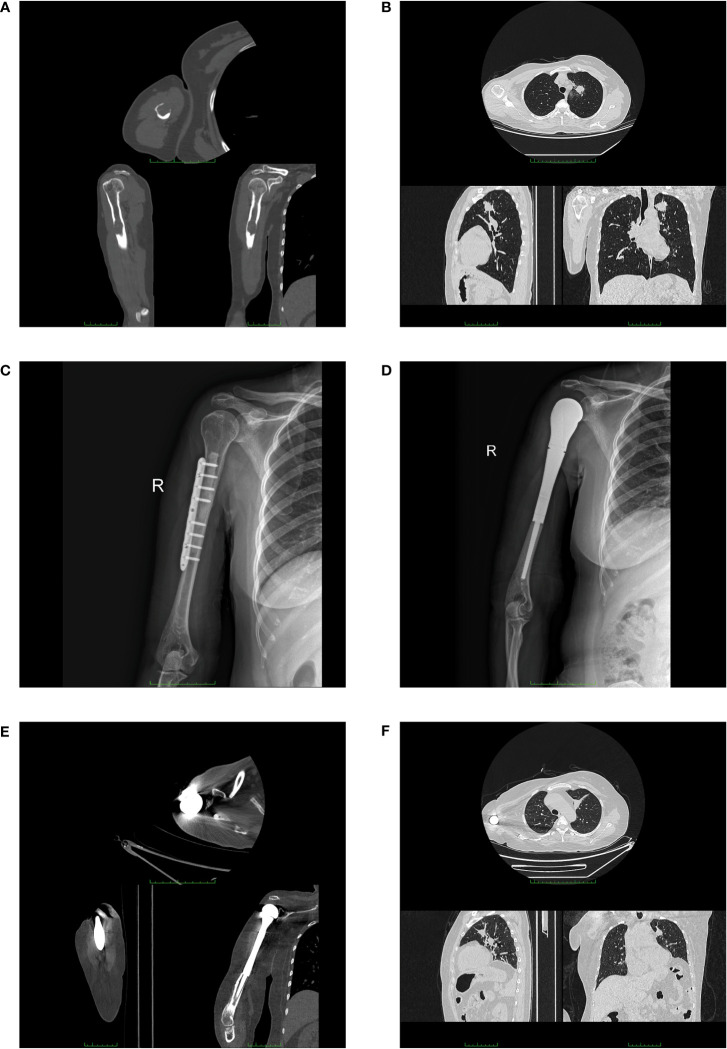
Imaging examination of the patient. Computed tomography images (upper image for axial, lower left for sagittal, and lower right for coronal, respectively) of the patient at the initial diagnosis (A, B). (A) CT scan showed a soft tissue mass (2.4 cm × 1.8 cm) and a pathologic fracture on the right humerus of the patient. (B) Chest CT showed a pulmonary mass in the left upper lobe. X-ray images after the first two surgeries (C, D). (C) X-ray image after the surgery for plate internal fixation on the right humerus. (D) X-ray image after the surgery for the right shoulder joint replacement. Computed tomography images (upper image for axial, lower left for sagittal, and lower right for coronal, respectively) before the surgery of shoulder disarticulation (E, F). (E) CT scan showed the prosthesis of patient exposed out of right humerus. (F) Chest CT presented stable disease in the primary lung tumor.
